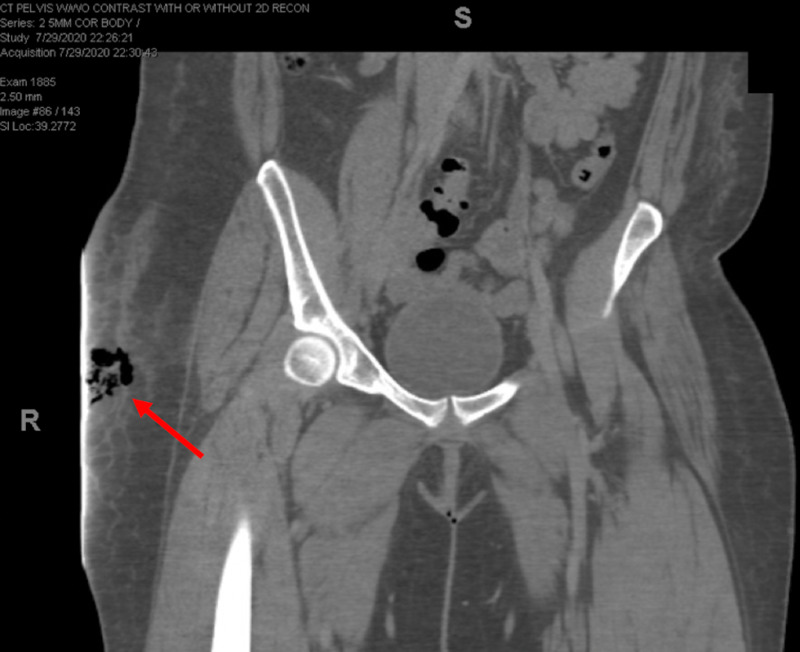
CT Pelvis demonstrating free gas in the patient's right hip soft tissue
